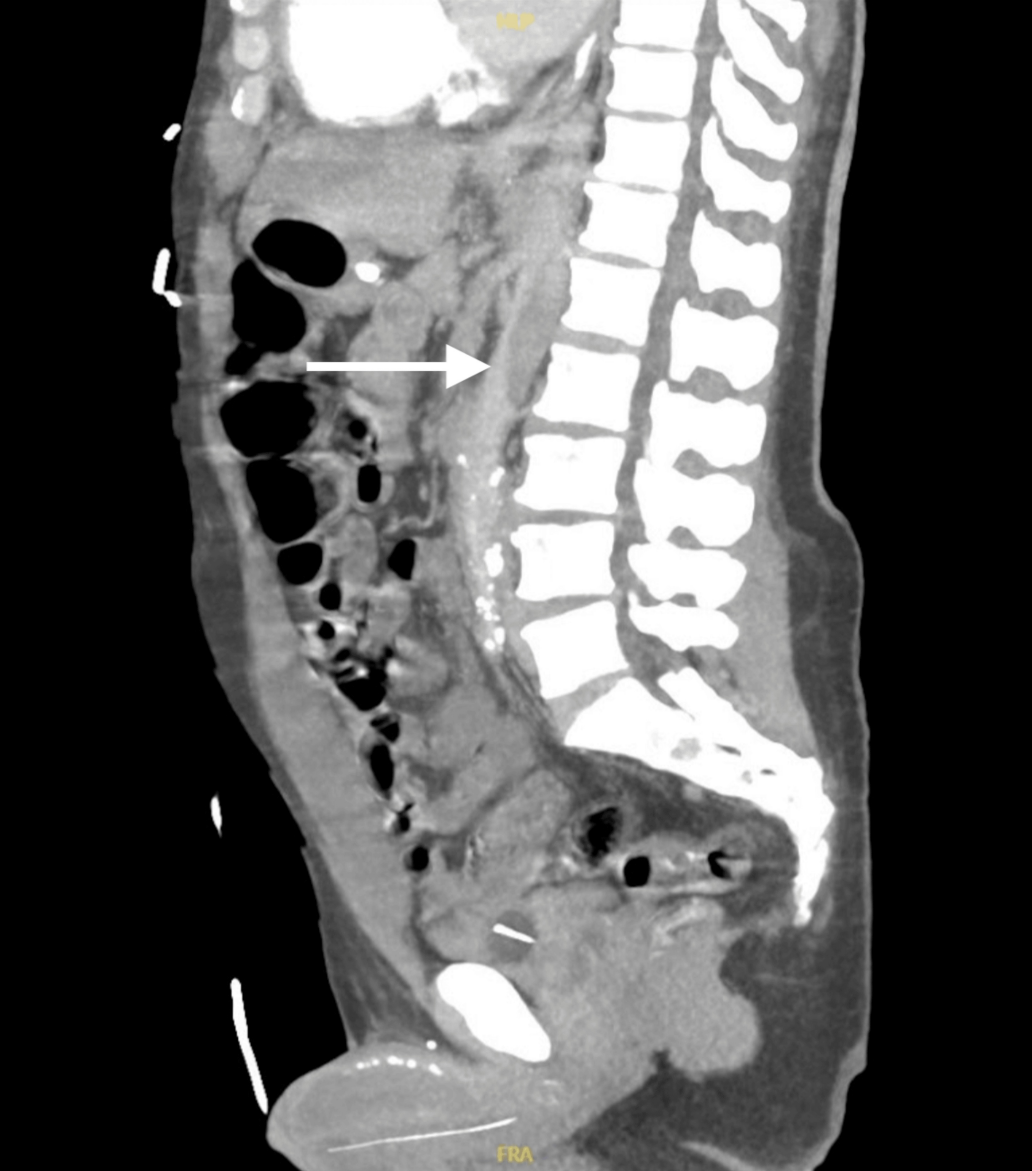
A sagittal section of a CT angiogram of the abdomen and pelvis showing an abdominal aortic dissection with extrinsic compression of the patent true lumen, as indicated by the arrows.
